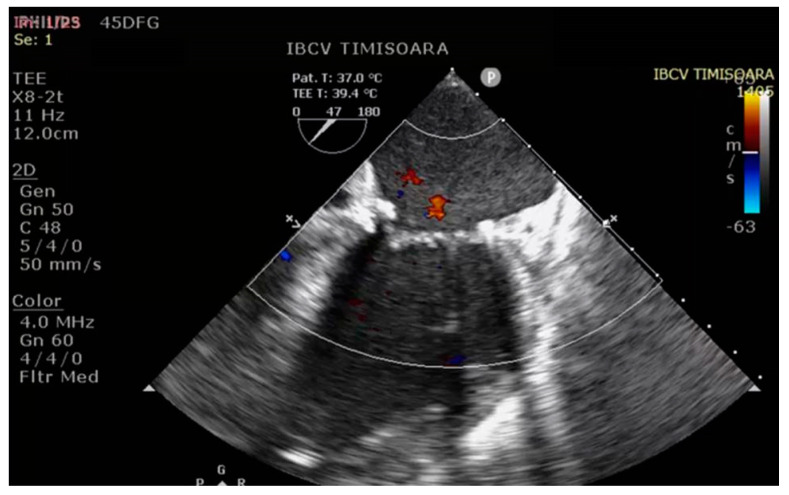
Post−operative aspect of the mitral valve (2D echocardiography).
